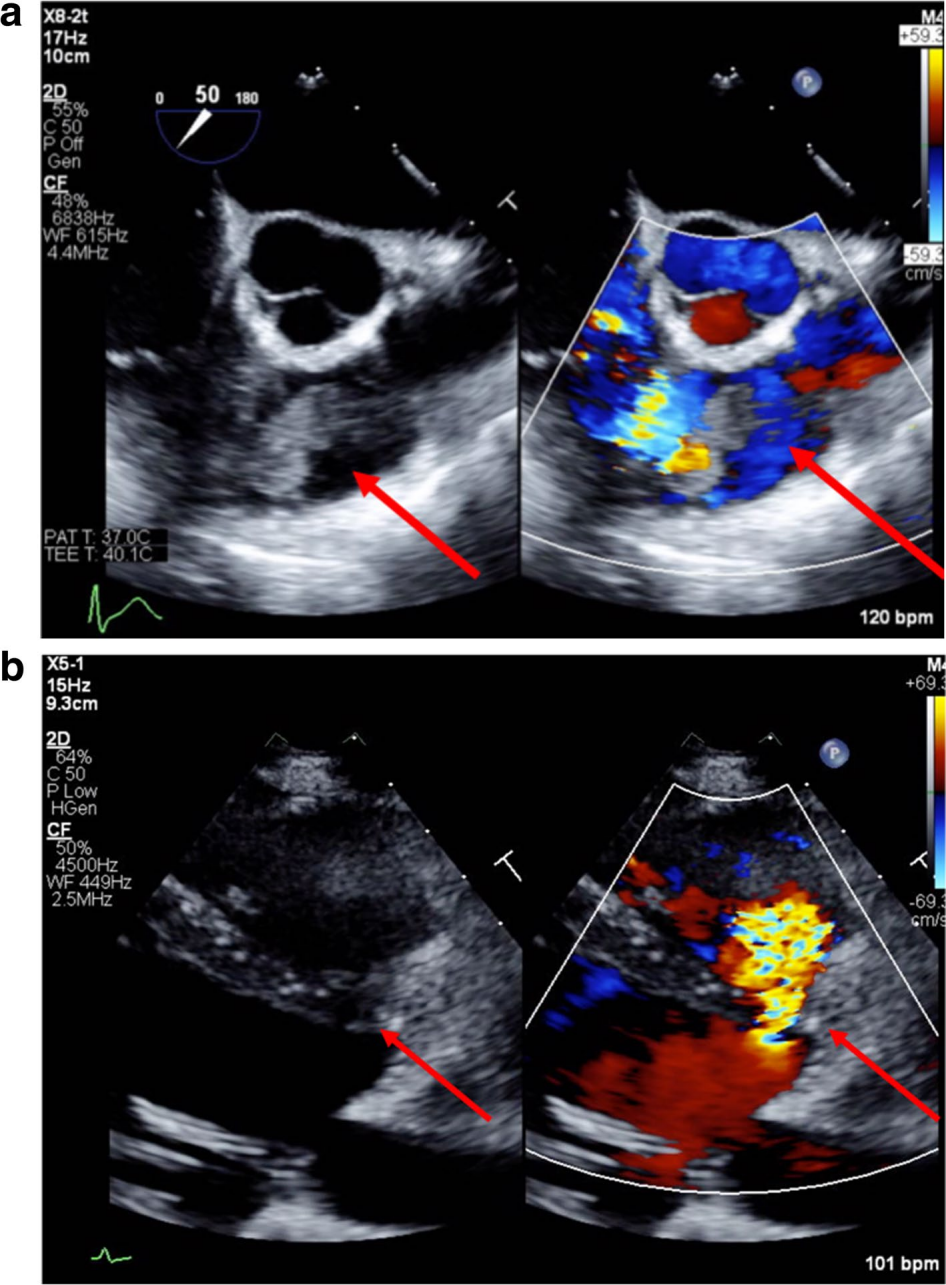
Preoperative transesophageal echocardiography. a The vegetation in the right ventricle is located close to the pulmonary valve. b The ventricular septal defect jet is directed to the right ventricular wall
